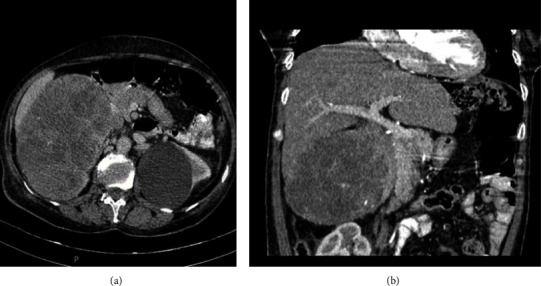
Abdominal CT scan with IV contrast. (a) Axial view of the heterogenous well-defined mass above the right kidney. (b) Coronal view of the adrenal mass effect on the IVC and pancreatic tail.
